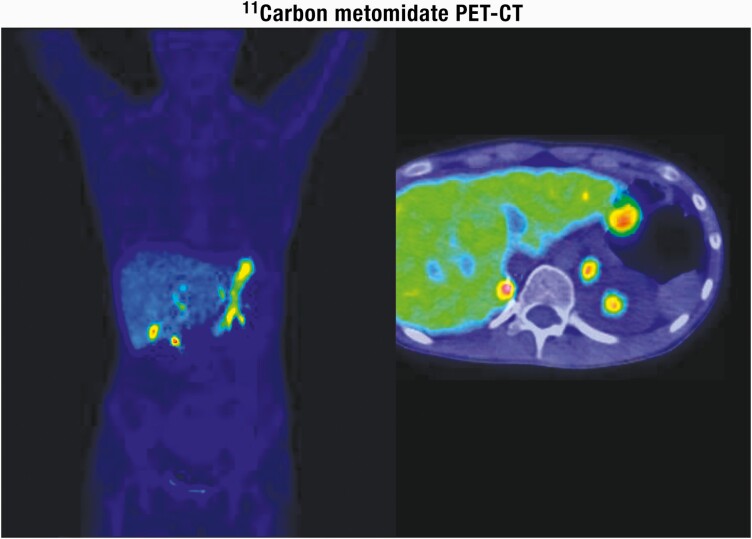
Carbon-11 metomidate PET-CT: Coronal PET maximum intensity projection and axial fused PET-CT images in a patient with a left adrenocortical carcinoma and an unsuspected tracer-avid right sided liver metastasis. Prominent physiological tracer uptake is present in the gastric wall and left renal collecting system. Images courtesy of Professor Anders Sundin, Consultant Radiologist, Uppsala University Hospital, Sweden.
